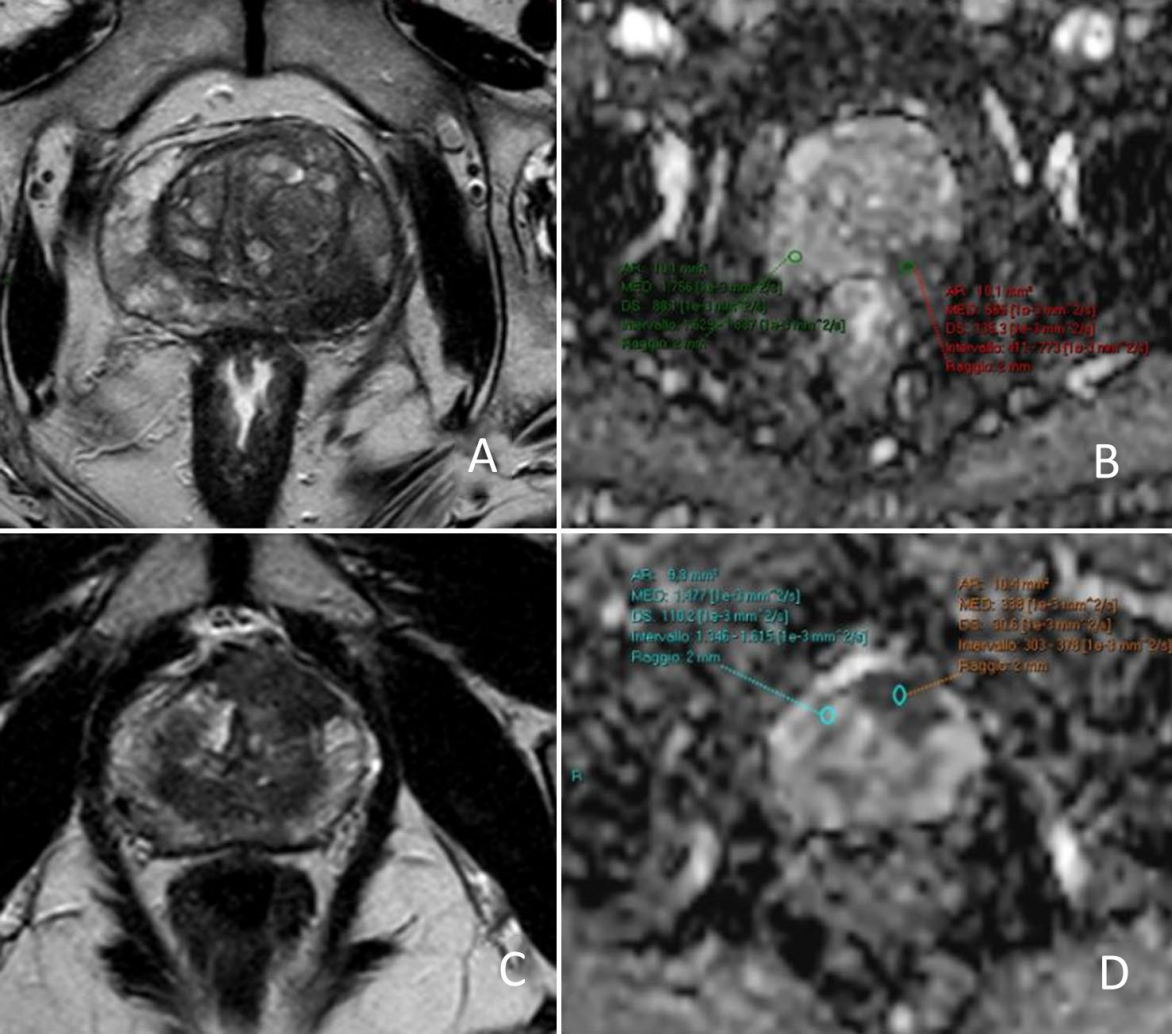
a, b ADC ratio calculation in a suspected PZ lesion in a 71 years old man. ADC ratio is 0.32 (565/1756). FPB later confirmed a PCa Gleason score 7 (4 + 3) in the area. c, d ADC ratio calculation in a suspected TZ lesion in a 70 year old man. ADC ratio was 0.22 (338/1477). FPB afterward confirmed a PCa Gleason score 8 (4 + 4) in the mpMRI signaled lesion. *ADC* apparent diffusion coefficient, *PZ* peripheral zone, *FPB* fusion prostate biopsy, *PCa* prostate cancer, *TZ* transitional zone, *mpMRI* multiparametric prostate MRI
